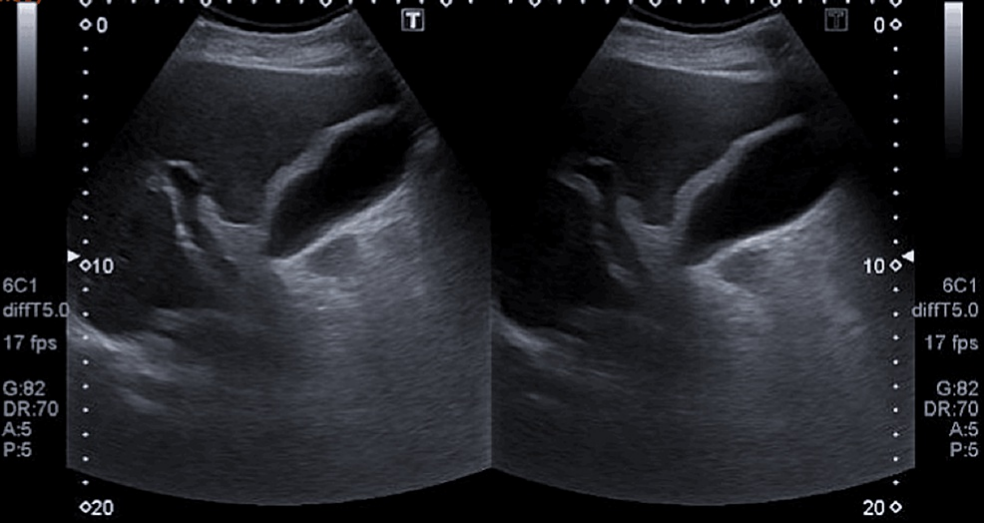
Abdominal ultrasound with a moderately distended gallbladder, admitting mild diffuse parietal thickening, without lithiasis or biliary sludge, findings compatible with alithiasic cholecystitis
